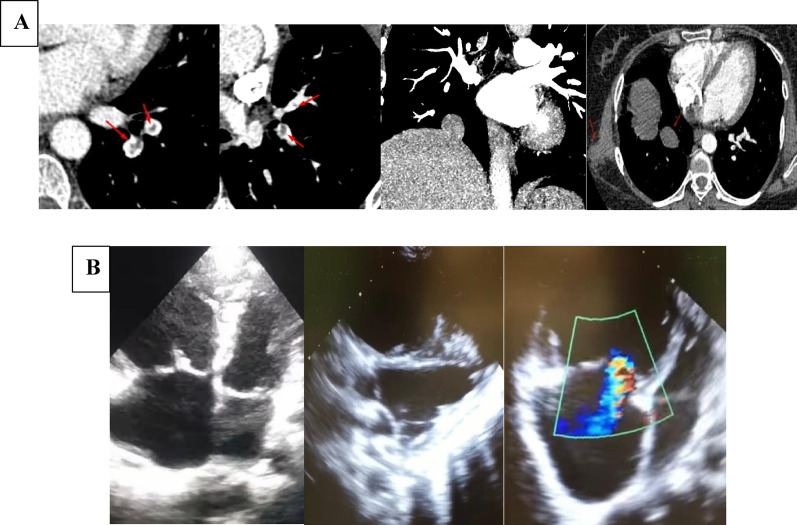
Case of 53-year-old male patient presenting with PE. A CTPA showing thrombo-embolism along the lobar branches of both pulmonary arteries, with extension along the segmental branches (on left side). (Red arrows). B TTE showing dilated RV and RA with moderate TR and flattened interventricular septum. *PE* Pulmonary Embolism; *CTPA* computed Tomography pulmonary Angiography; *TTE* Transthoracic Echocardiography; *RV* Right Ventricle; *RA* Right Atrium; *TR* Tricuspid Regurgitation
